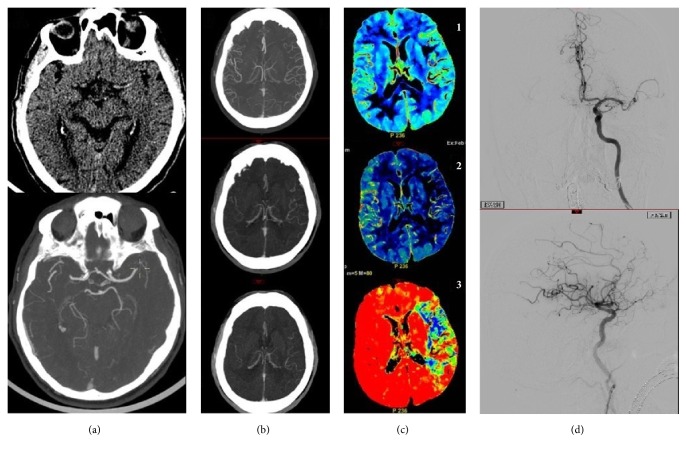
82-year-old patient, smoker, presenting at 2 hours from symptoms onset with an NIHSS score of 20. (a) CT shows left hyperdense MCA, CTA absence of flow in the same MCA region. (b) Multiphase CTA shows good collaterals in the arterial phase and two venous phases of acquisition. (c) CTP shows mismatch between the large area on MTT (3) and the small infarct core on CBV (1). (d) Angiography shows an occluded MCA with perfect recanalization (TICI 3).
